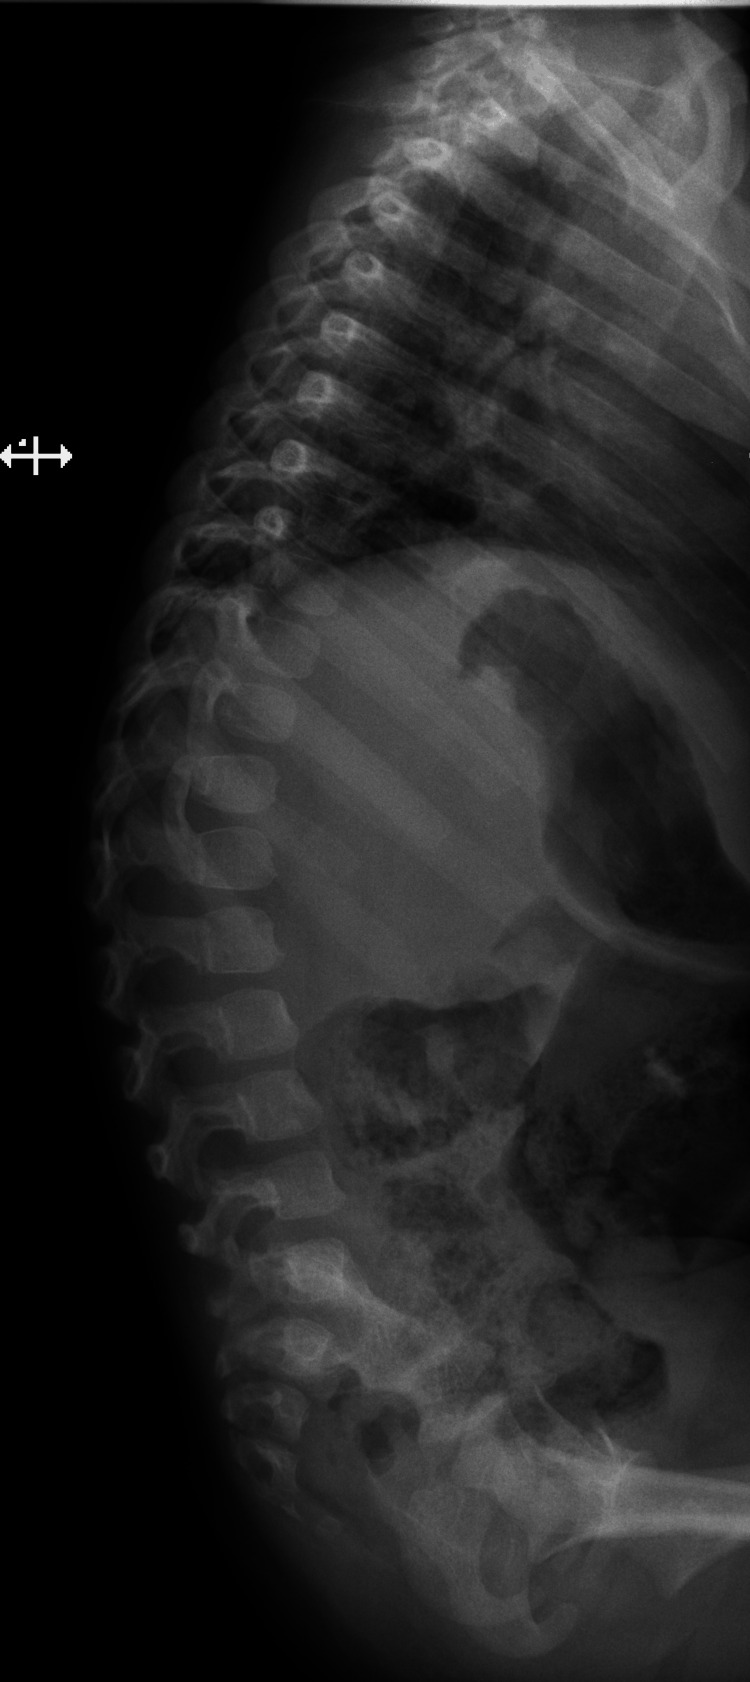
Spine radiography at 27 months (lateral view). Absence of natural spine curves, with a mid-thoracolumbar kyphosis (mostly positional).
